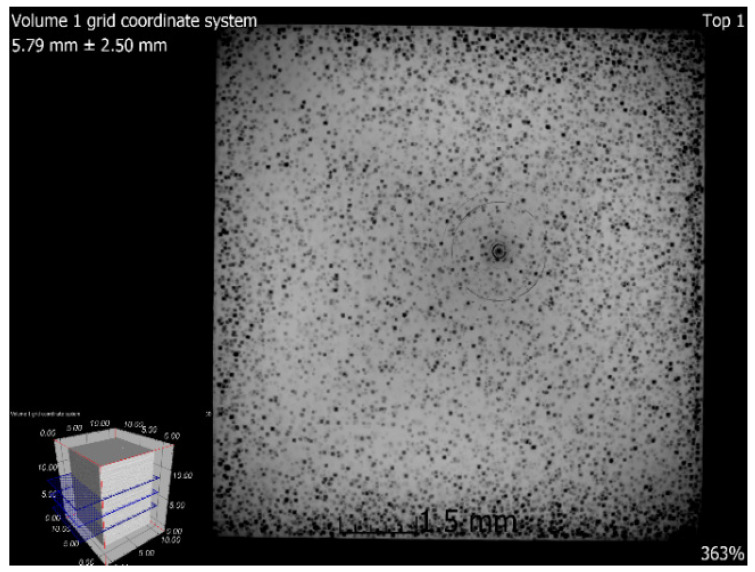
Tomographic CT sections of sample 5 after SLM (XY plane).
